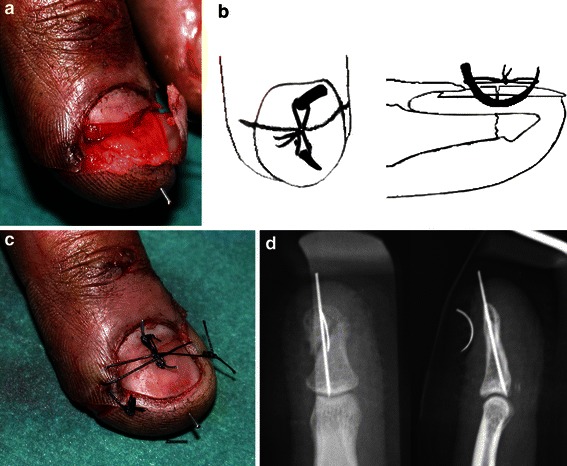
a Transversal nail-bed lesion, b nail haubanage, c postoperative nail haubanage, d postoperative nail haubanage X-ray
